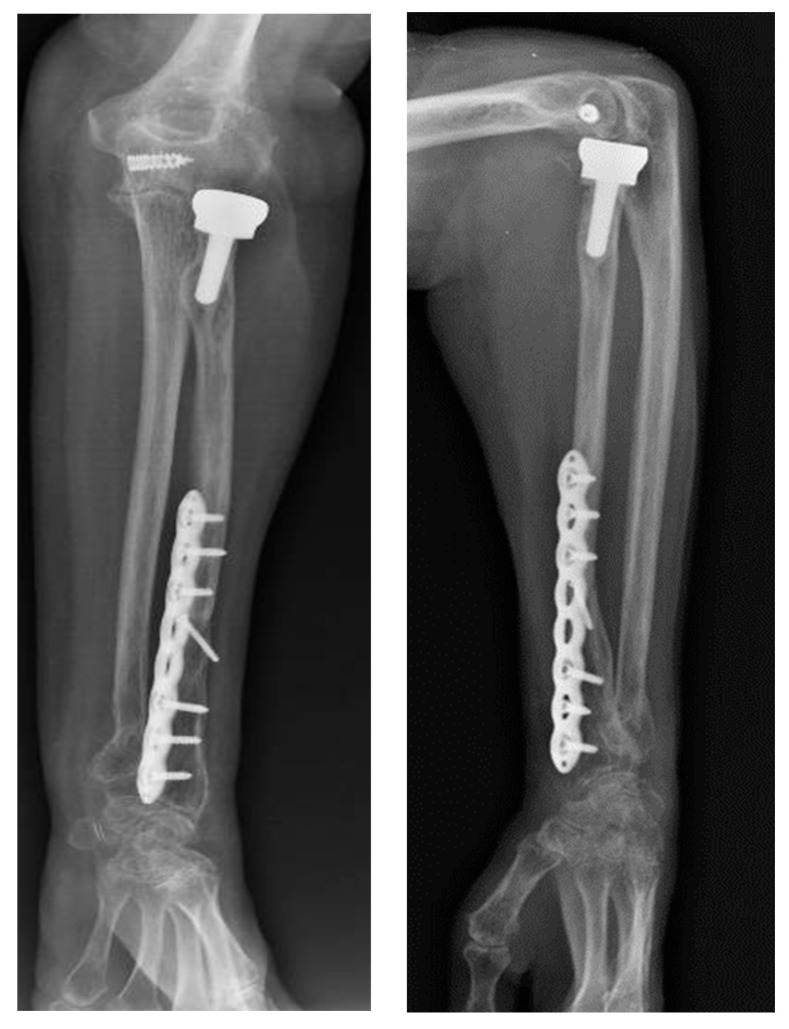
Two-year follow-up X-rays.
